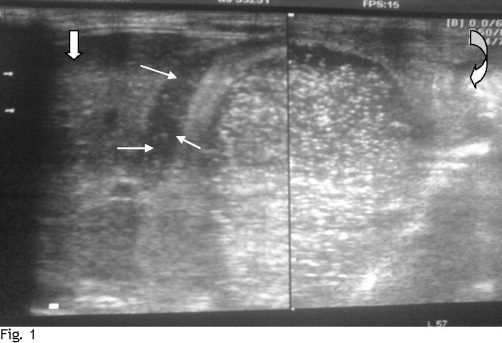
Transabdominal sonograms showing a well-circumscribed giant meconium cyst. Note the multiple brightly echogenic internal echoes and layering (image to the left). The deuodenum (short arrows) and liver (block arrow) are normal. The compressed urinary bladder is indicated by curved arrow.
